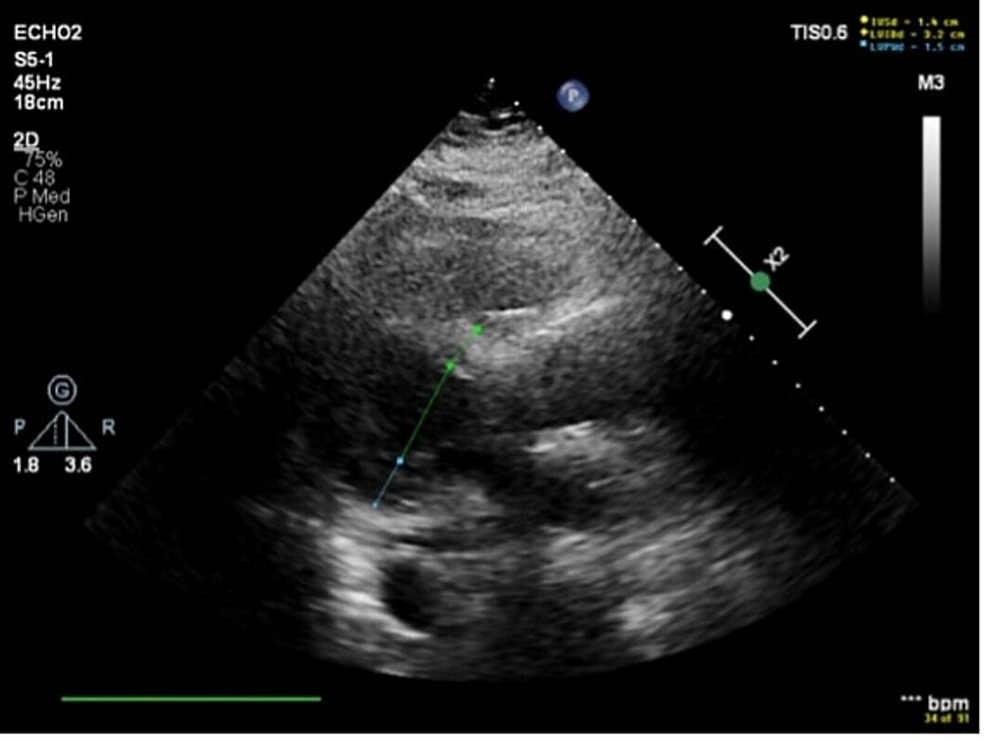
Limited 2D Echocardiogram showing Left Ventricular Internal End-Diastolic Diameter (LVIDd) of 3.2 cm. (Parasternal long axis)
